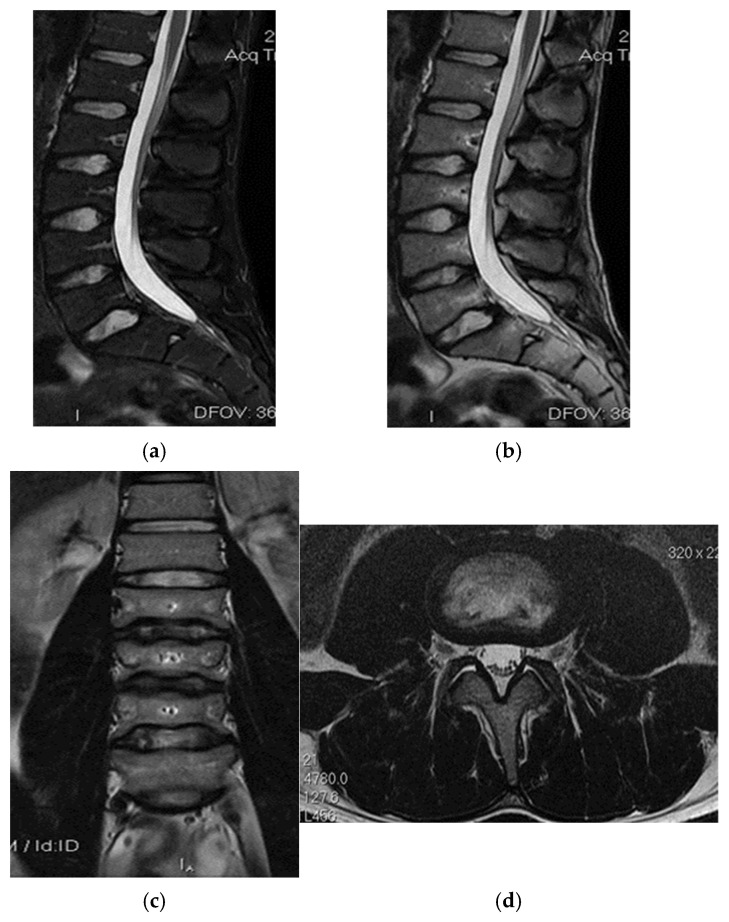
(a) Spinal MRI: sagittal T2 fat sat acquisition, (b) spinal MRI: sagittal T2 acquisition, (c) spinal MRI: coronal T2 acquisition, (d) spinal MRI: axial T2 acquisition.
